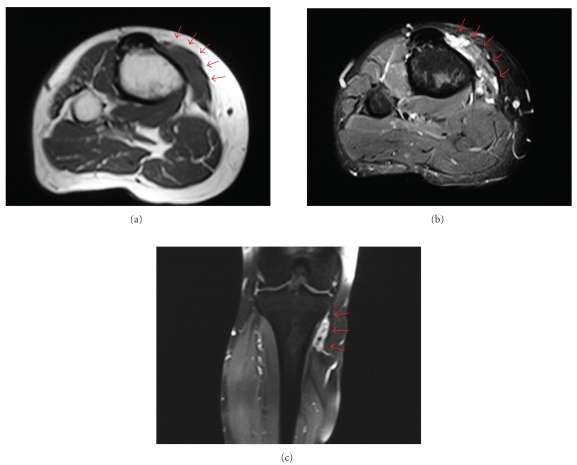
MRI with (a) axial T1 (TR 602, TE 40), (b) axial short tau inversion recovery (STIR; TR 4910, TE 40), and (c) contrast enhanced coronal T1 fat saturated(TR 540, TE 13) sequences showed a heterogeneous soft tissue mass at the insertion of pes anserinus conjoint tendon with peripheral and septal enhancement. The decreased interspersed signal on all images was due to hemosiderin deposits. There was no evidence of bone or joint involvement.
